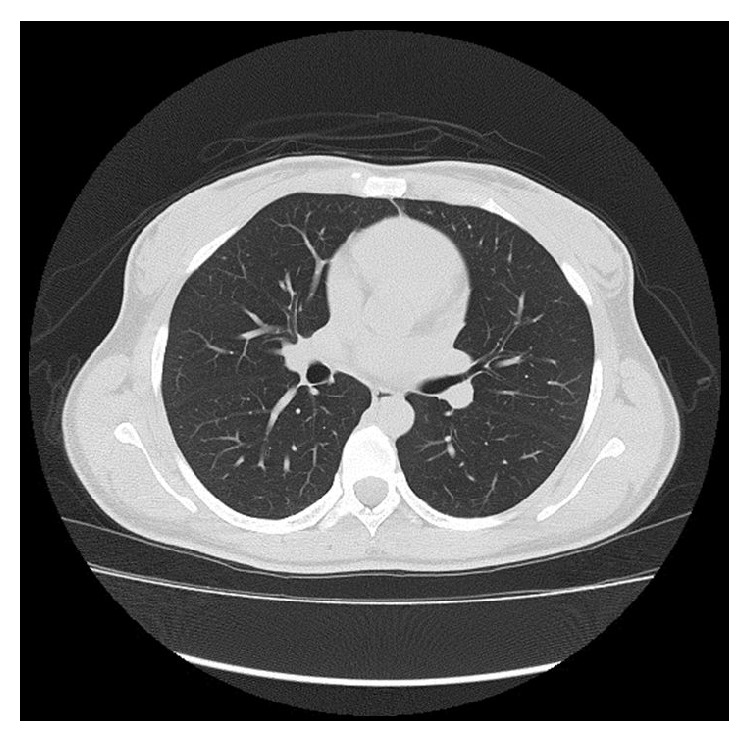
Contrast-enhanced computed tomography on 1-month follow-up demonstrating complete resolution of the parenchymal opacifications.
